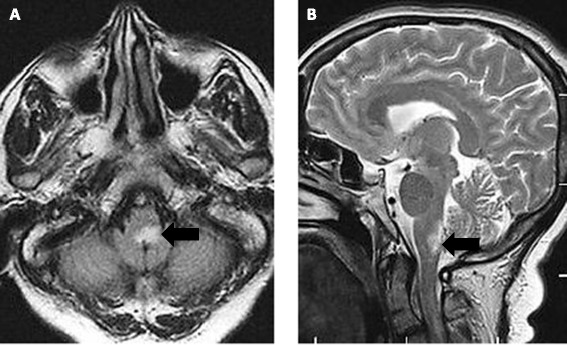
Axial (A) and sagittal (B) T2-weighted FLAIR magnetic resonance imaging showing high signal intensity lesion in the dorsal medulla corresponding to the area postrema (arrows).
